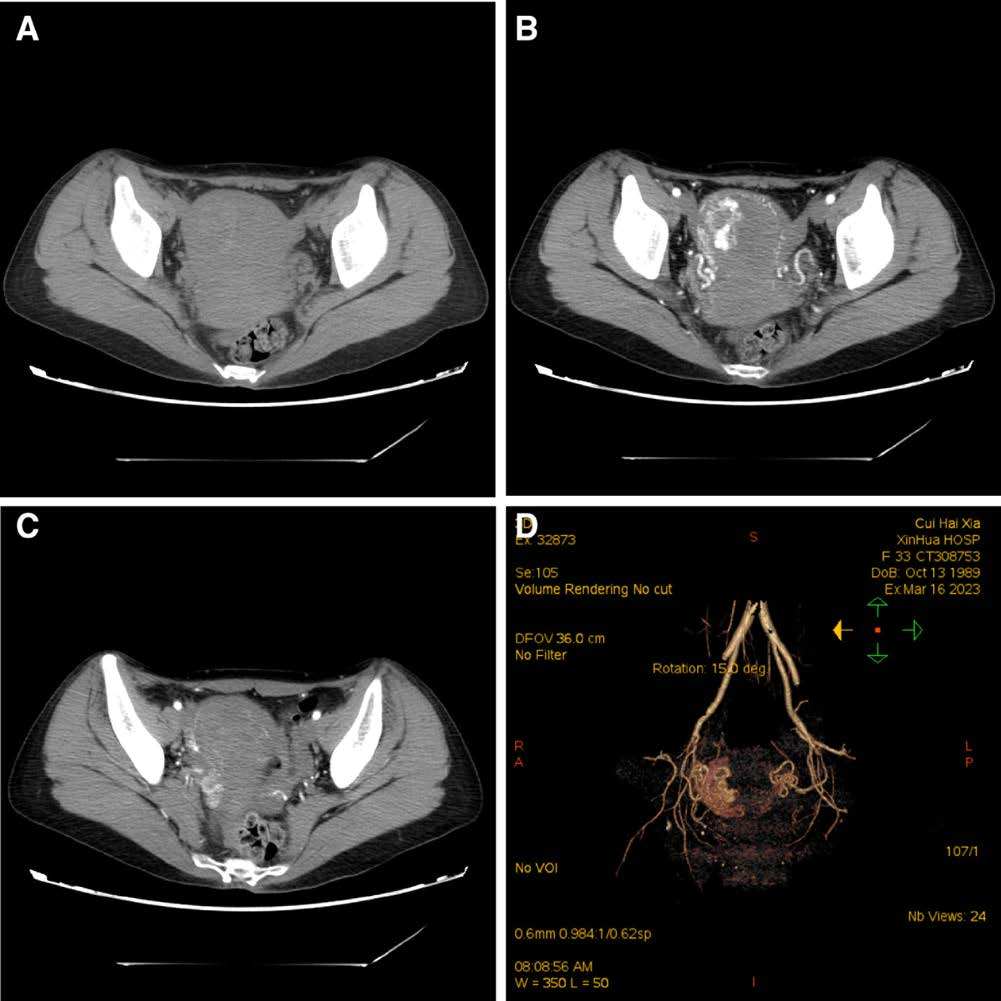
Computed tomography (CT) and CT angiography (CTA) images show uterine arteriovenous fistula (UAVF). Plain CT scan (A) shows that the lesion was located in the right uterine horn, and patchy hyperdensity was seen within it. An enhanced CT image (B) shows uneven and markedly enhanced lesions, similar to vascular enhancement. An enhanced CT image (C) shows that the right parametrial vein appears earlier than the contralateral side. CTA reconstruction (D) shows increased thickening and tortuosity of the right uterine artery branches, and multiple peripheral draining veins have developed.
